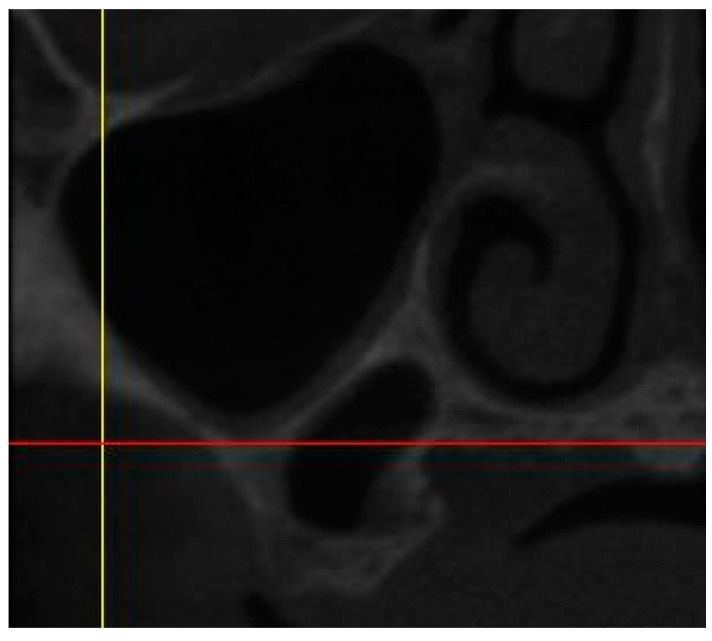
CBCT image showing the presence of sinus septum.
